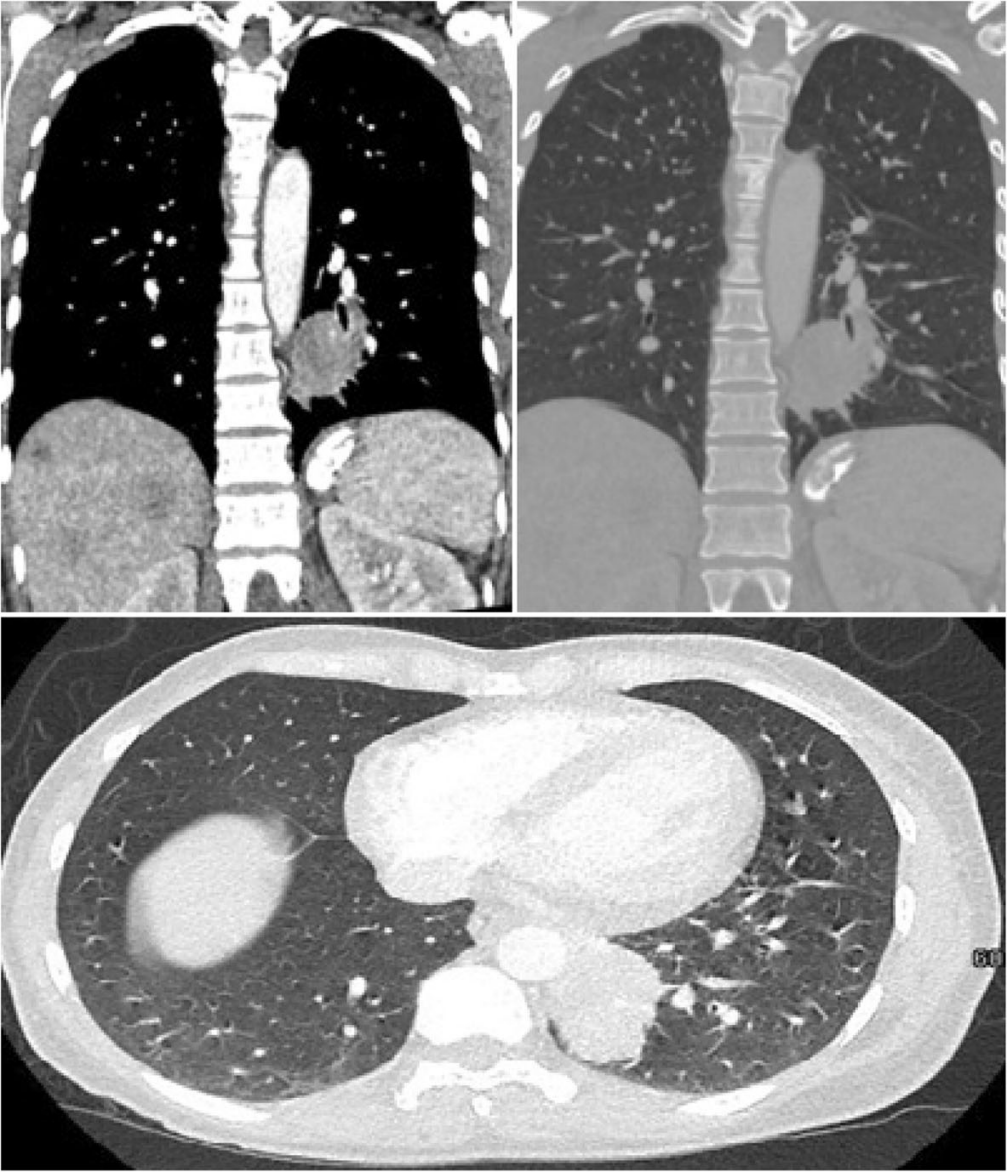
Thorax computed tomography. Solid mass with heterogenic enhancement, with well-defined intrapulmonary spiculated contours in the anteromedial segment of the lower lobe of the left lung of 4.7 × 3.3 cm (anteroposterior × transverse)
